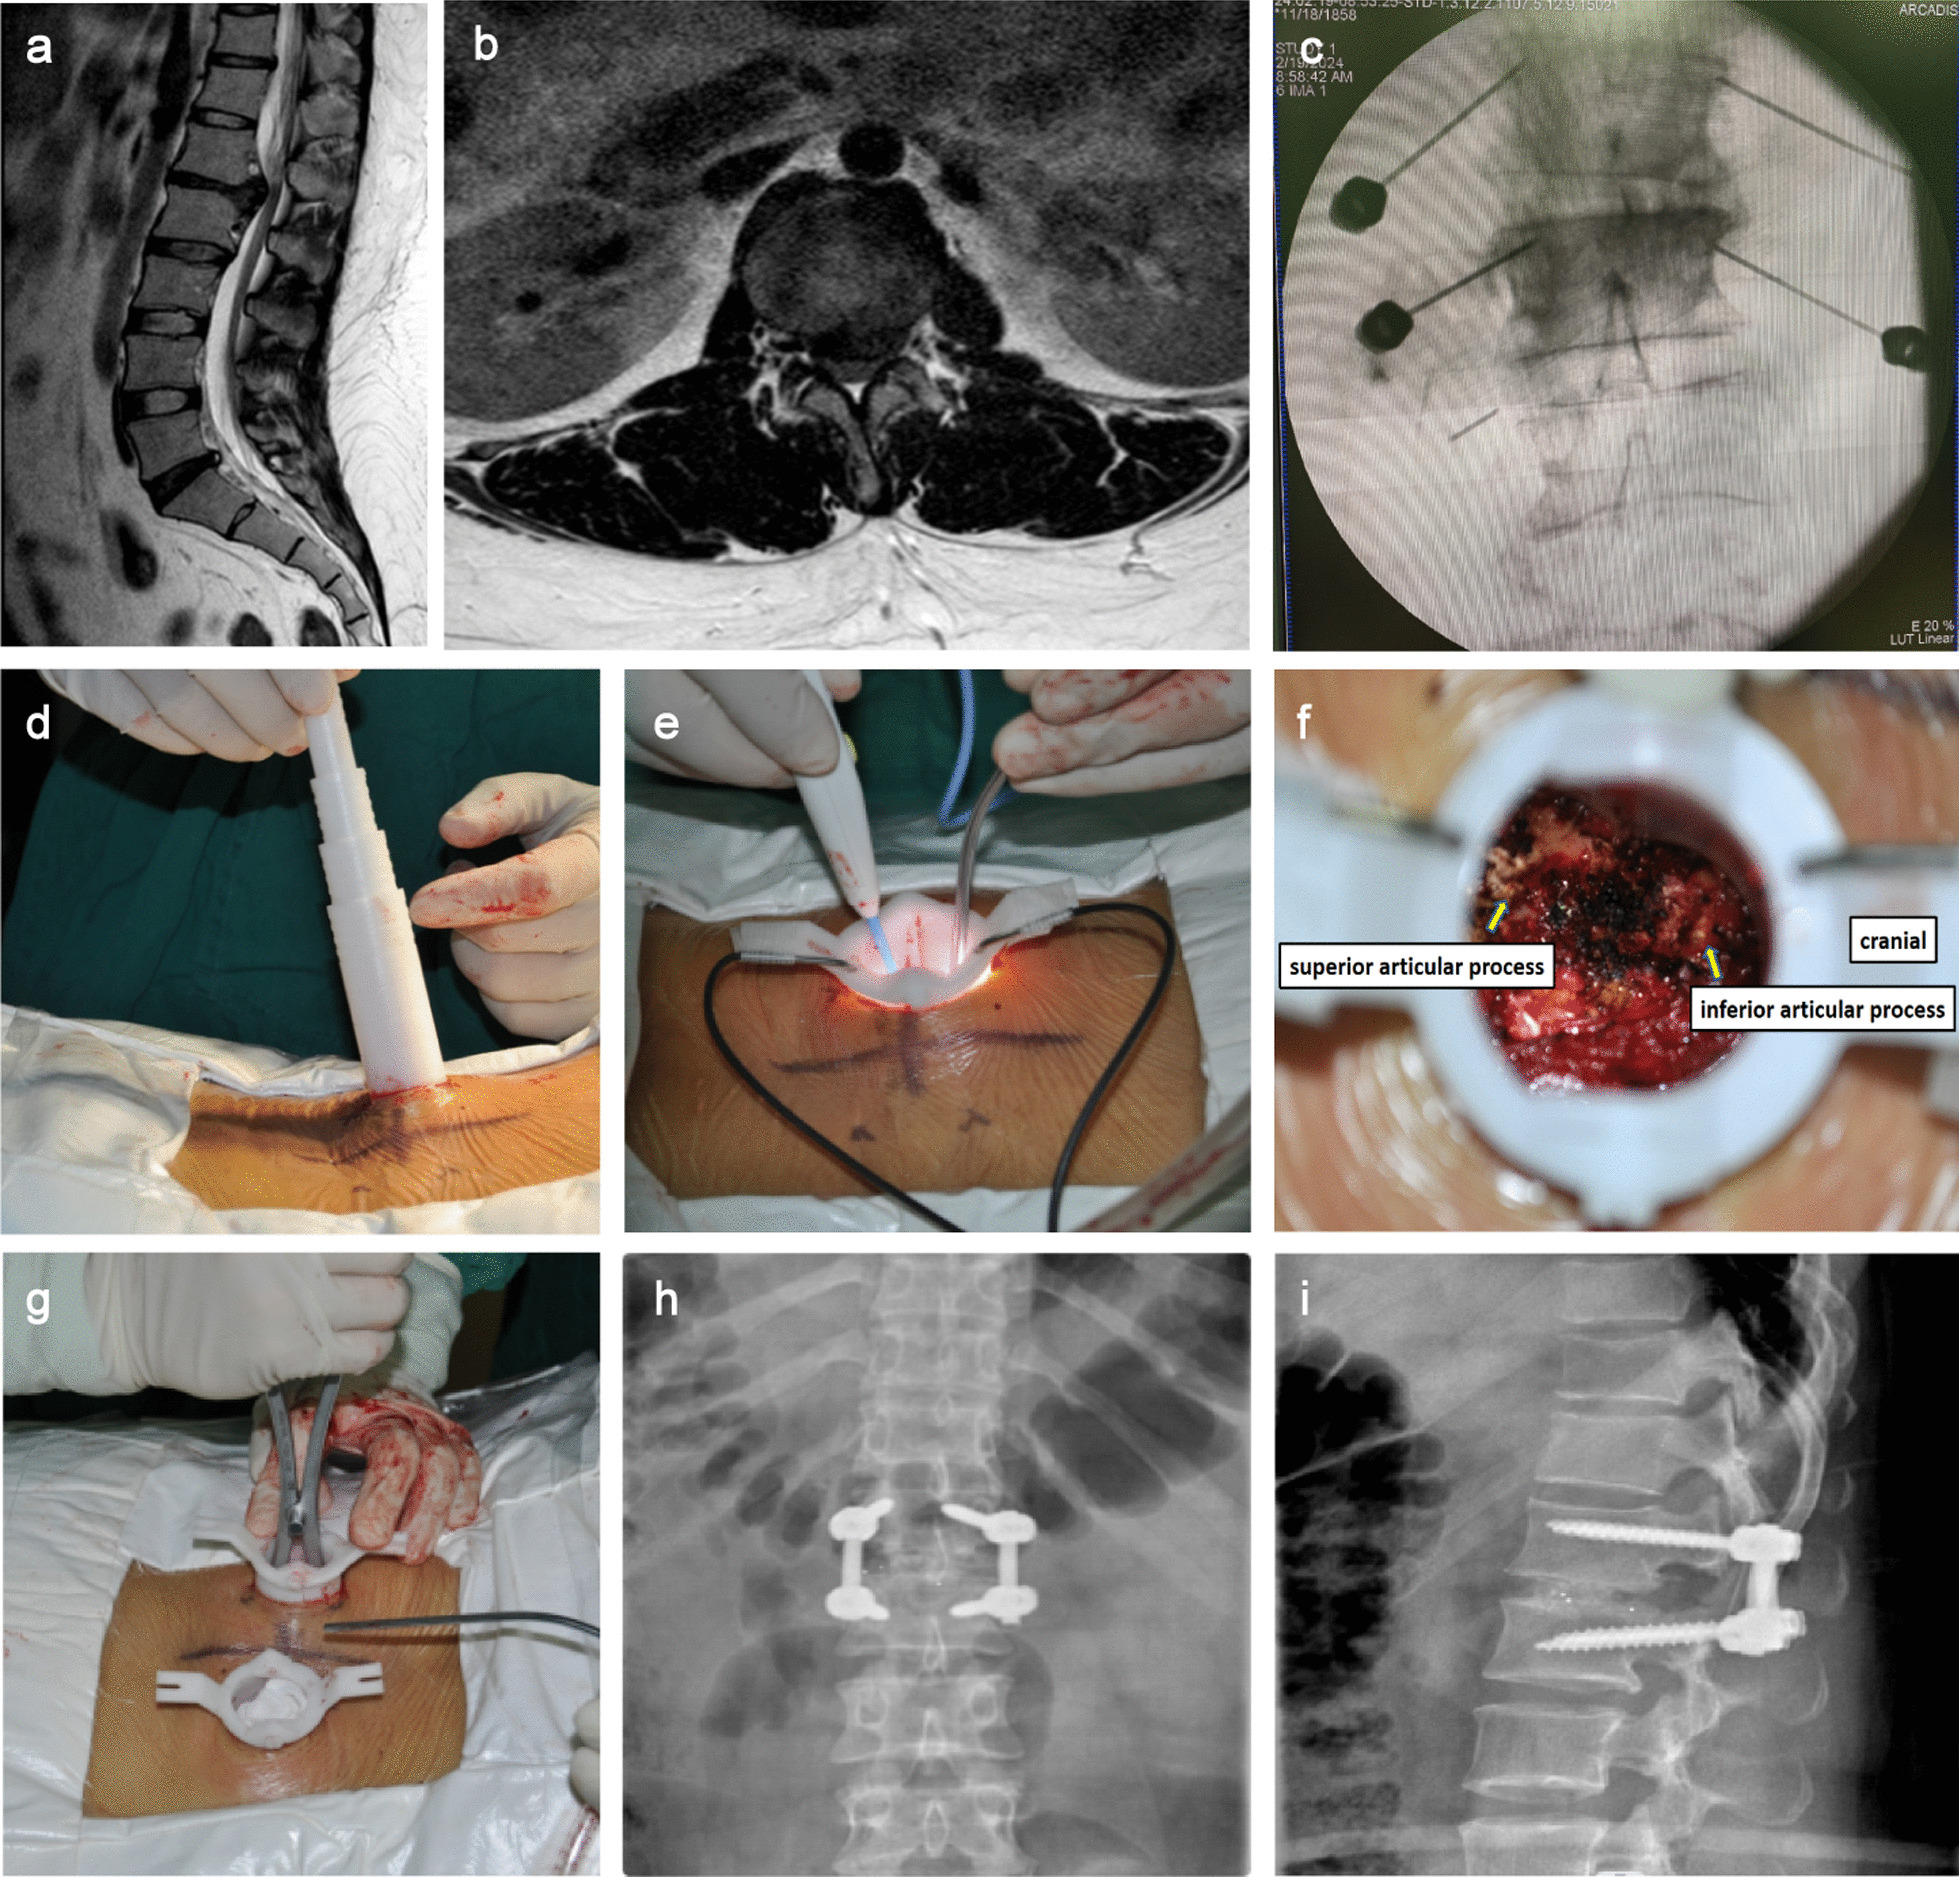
Patient, female, 26 years old, upper lumbar disc herniation undergoing MIS-TLIF surgery (L1-L2). a Patient's preoperative sagittal MRI. b Patient's preoperative horizontal MRI. c Intraoperative positioning of the long needle puncture. d Placement of disposable working tube. e the light source was placed and the soft tissues around the facet joint were eliminated. f Surgical view in the working tube. g On the symptomatic side, the same method was used as on the contralateral side to place the tube, prepare the nail paths, and seal it with bone wax. h, i It was satisfactory to determine the position of the rod and cage on postoperative fluoroscopy
